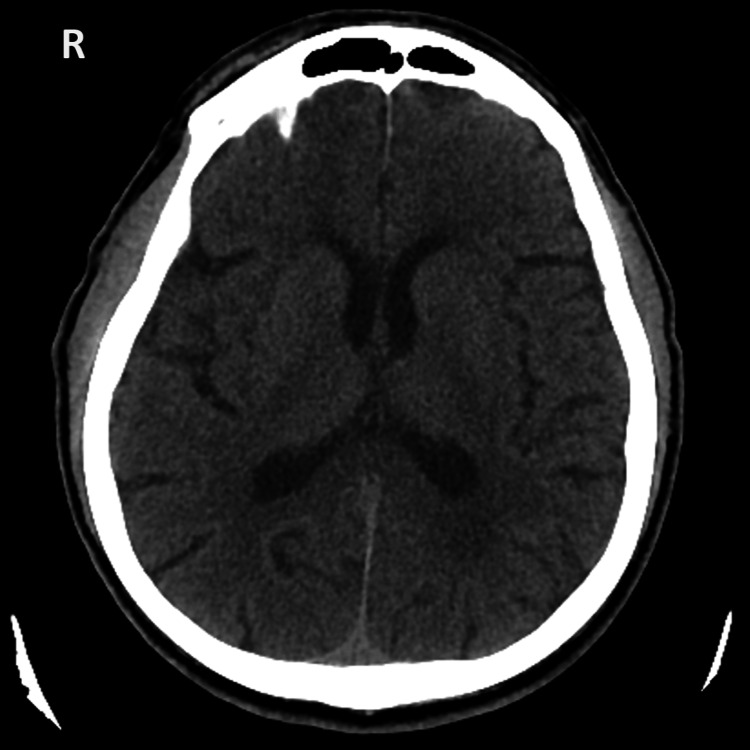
Admission head CT scan. Axial view showing no signs of lesions.
